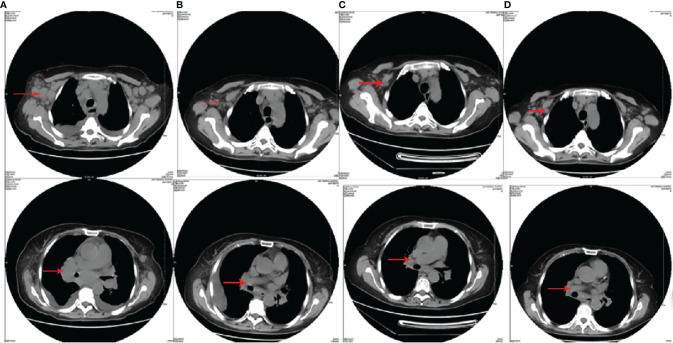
The chest CT images of patient 1. (A) The chest CT before CHOPE (bilateral axillary, mediastinum, bilateral hilar, and bilateral cervical lymphonodus were enlarged); (B) the chest CT after three cycles of CHOPE, before CAOLD (mediastinal and hilar lymphonodus enlarged again; the size of axillary lymphonodus was the same as before); (C) the chest CT after two cycles of CAOLD (mediastinal and hilar lymphonodus were significantly reduced); (D) the chest CT after five cycles of CAOLD (mediastinal and hilar enlarged lymphonodus almost disappeared).
